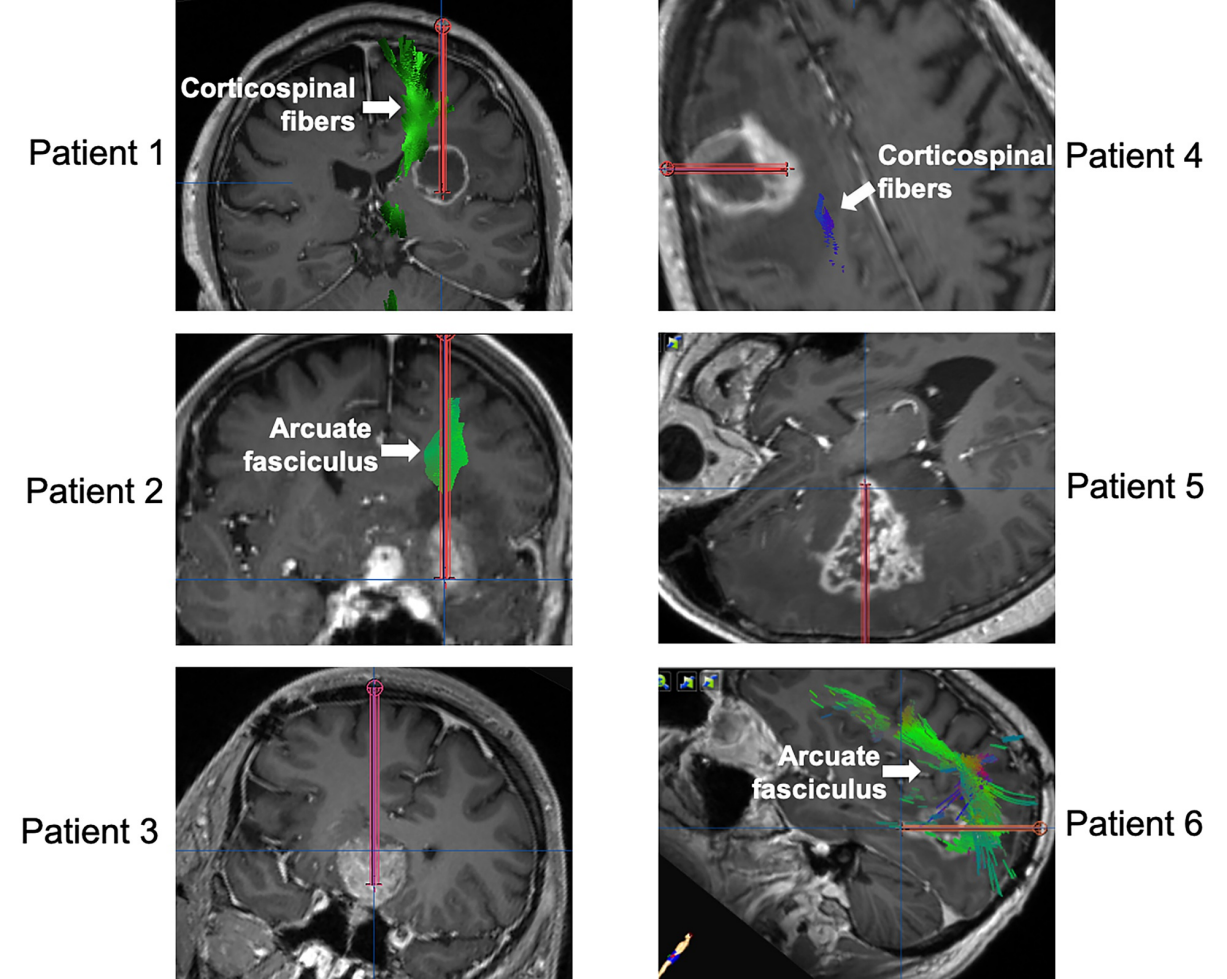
Preoperative imaging and planned trajectories. T1-weighted post-gadolinium images (“inline” or “trajectory” views) show the tumor targets and planned trajectories for each patient included in this study. Tractography identifies relevant fiber tracks in each case. For patient 3, the optic apparatus (chiasm and tracts) is not visible on this view, while for patient 5, the corticospinal tract is not visualized in this image (but can be appreciated in Figure 1).
